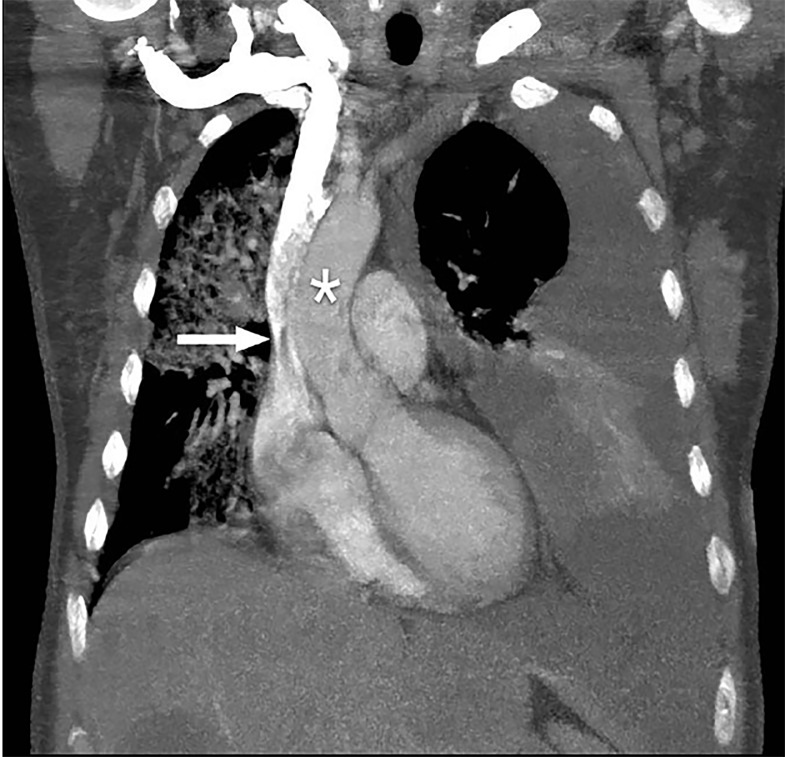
Thoracic computed tomography angiography coronal view with rightward mediastinal shift (*) and compression of superior vena cava (white arrow).
